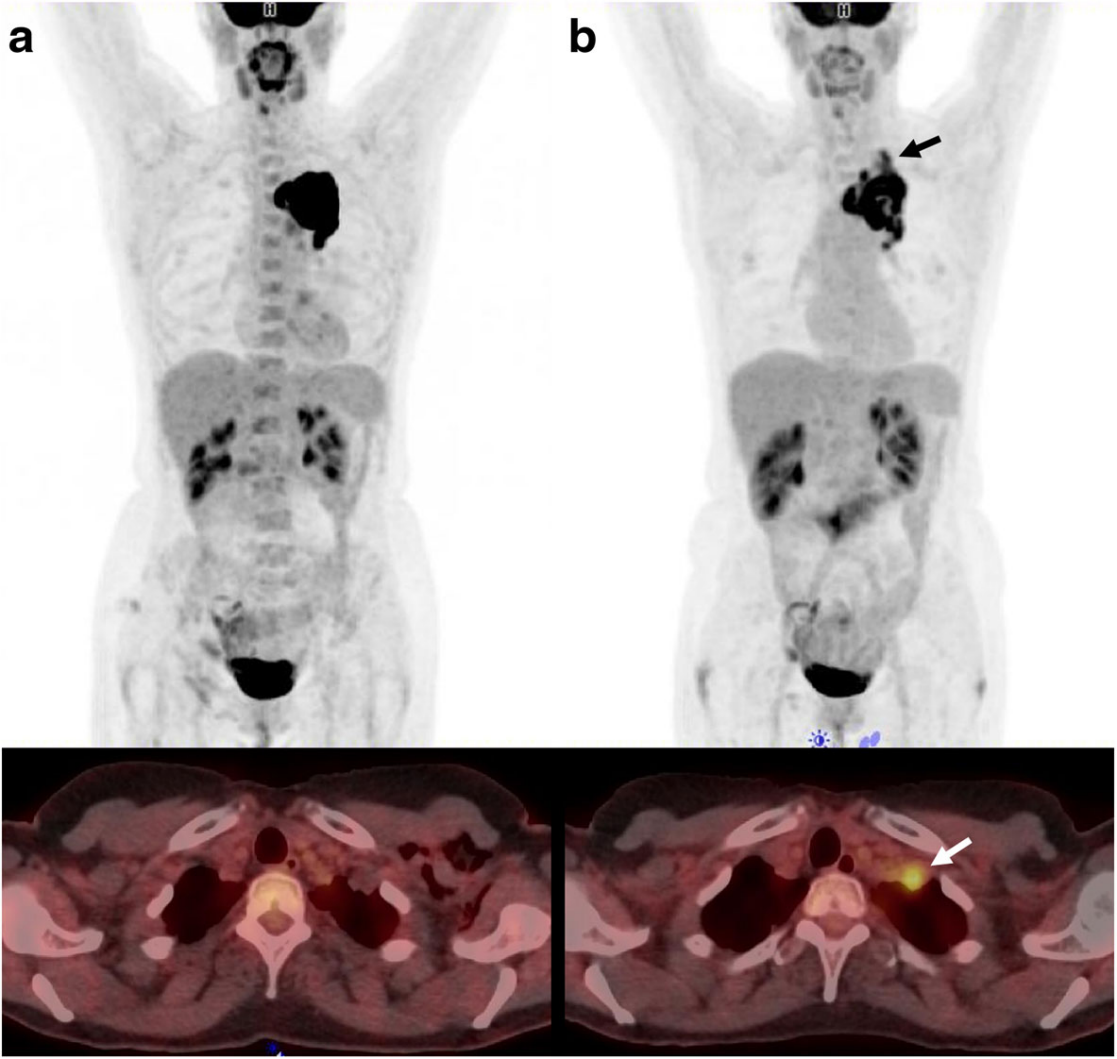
A typical case of disagreement between observers. a is the baseline and b is follow-up. On the *bottom*, one of the foci with uptake on follow-up is presented. In general, all observers found that a decrease in FDG-uptake was seen on follow-up (b), but three observers found that new areas with high FDG-uptake represented new malignant lesions (PMD) and five observers evaluated PMR, indicating the foci most likely to be benign. All observers reported a 51 % SULpeak decrease
